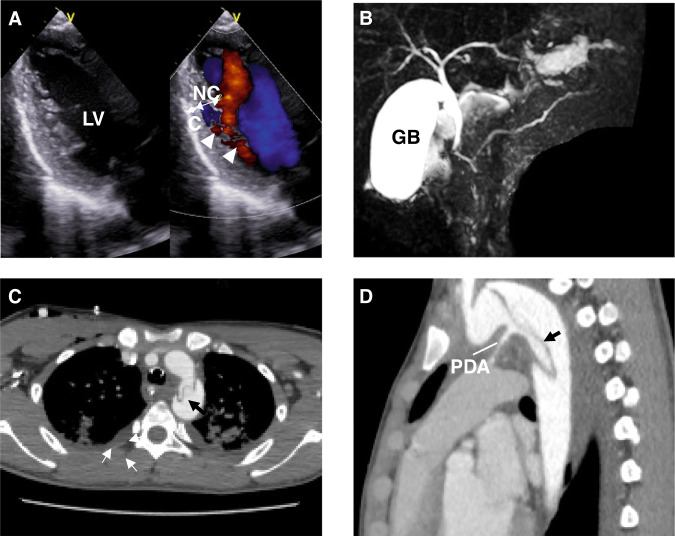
Echocardiography and magnetic resonance cholangio-pancreatography (MCRP) of the patient with the *ACTA2* G148R variant. A An echocardiogram showing the left ventricular long-axis view during diastole. The ventricular wall of the left ventricle exhibits an outer compacted layer (C) and an inner non-compacted trabecular layer (NC), with deep recesses formed by the trabeculations (NC/C ratio 2.0). Color Doppler imaging shows blood infiltrating into the spaces between the trabeculae (arrowheads). B On MRCP, the main pancreatic duct exhibits a reverse Z-shape (arrows) in the head of the pancreas region. C, D Chest magnetic resonance imaging shows the descending aortic deviation. LV left ventricle, C compacted layer, NC non-compacted layer, GB gallbladder, PDA patency of the ductus arteriosus
